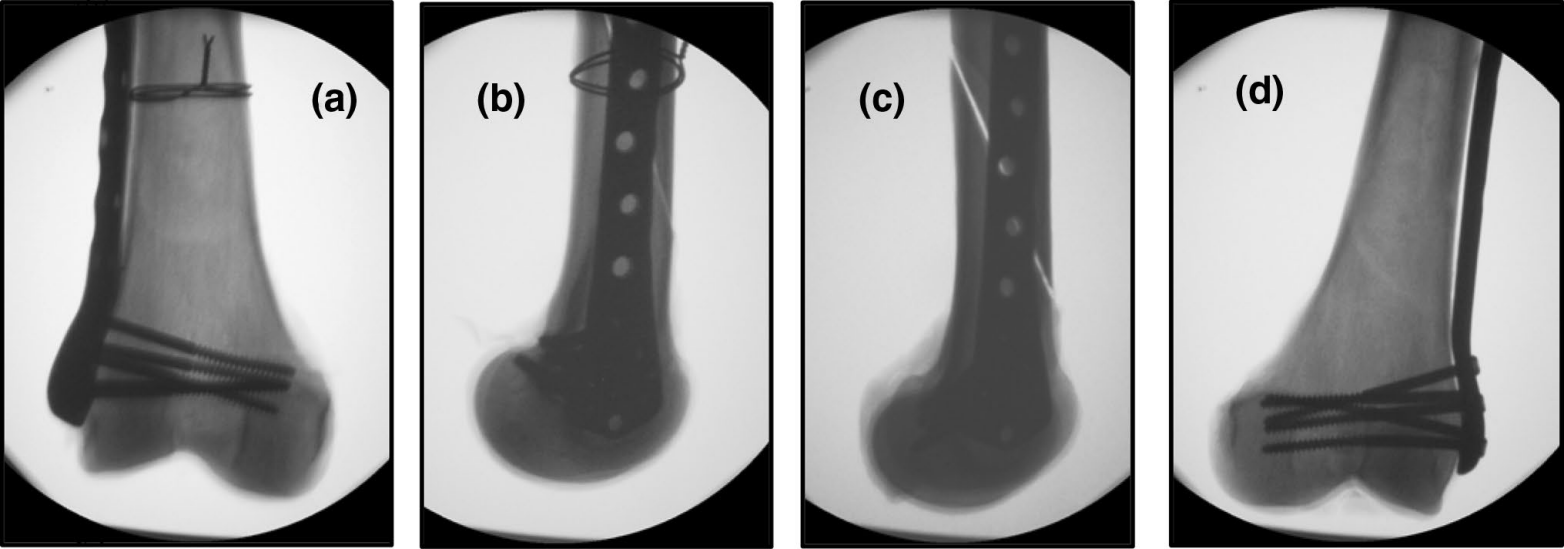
Typical postoperative X-ray pictures showing a pair of samples with an NCB-DF plate osteosynthesis and an additionally attached double-looped wire cerclage in the right femur (a and b) (the same femur is shown in Fig. 2). The left femur only received fixation with an NCB-DF plate (c and d)
